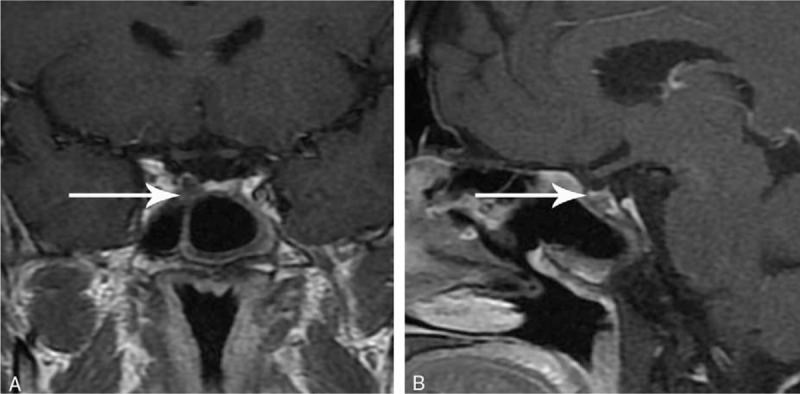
Postoperative MRI characteristics of the pituitary lesion: (A) coronal-enhanced T1WI, (B) sagittal-enhanced T1WI. MRI = magnetic resonance imaging, T1WI = T1-weighted image.
